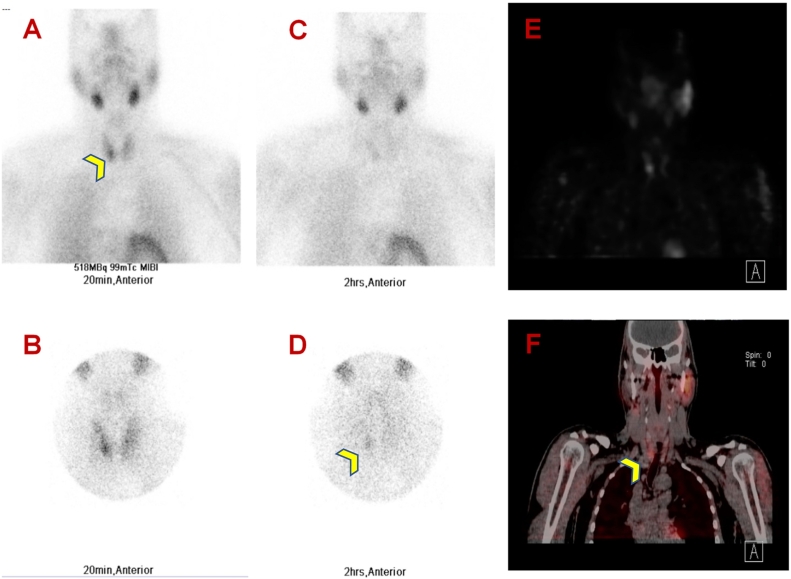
Parathyroid 99m Tc-sestamibi scintigraphy SPECT/CT. Early images (A-B) revealed tracer uptake in the thyroid tissues with a more intense uptake in a small focus related to the lower pole of right thyroid lobe (arrowhead); Late images (C-D) revealed physiological tracer washout from thyroid tissues but retained activity in the above-described focus; small focal radiotracer uptake (E-F) at the right lower pole of the thyroid gland, consistent with an active right inferior parathyroid adenoma.
